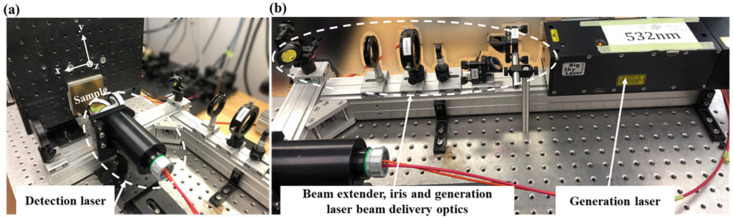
Laser ultrasonic experimental setup: (a) generation laser and optical path unit, and (b) detection laser and sample.
